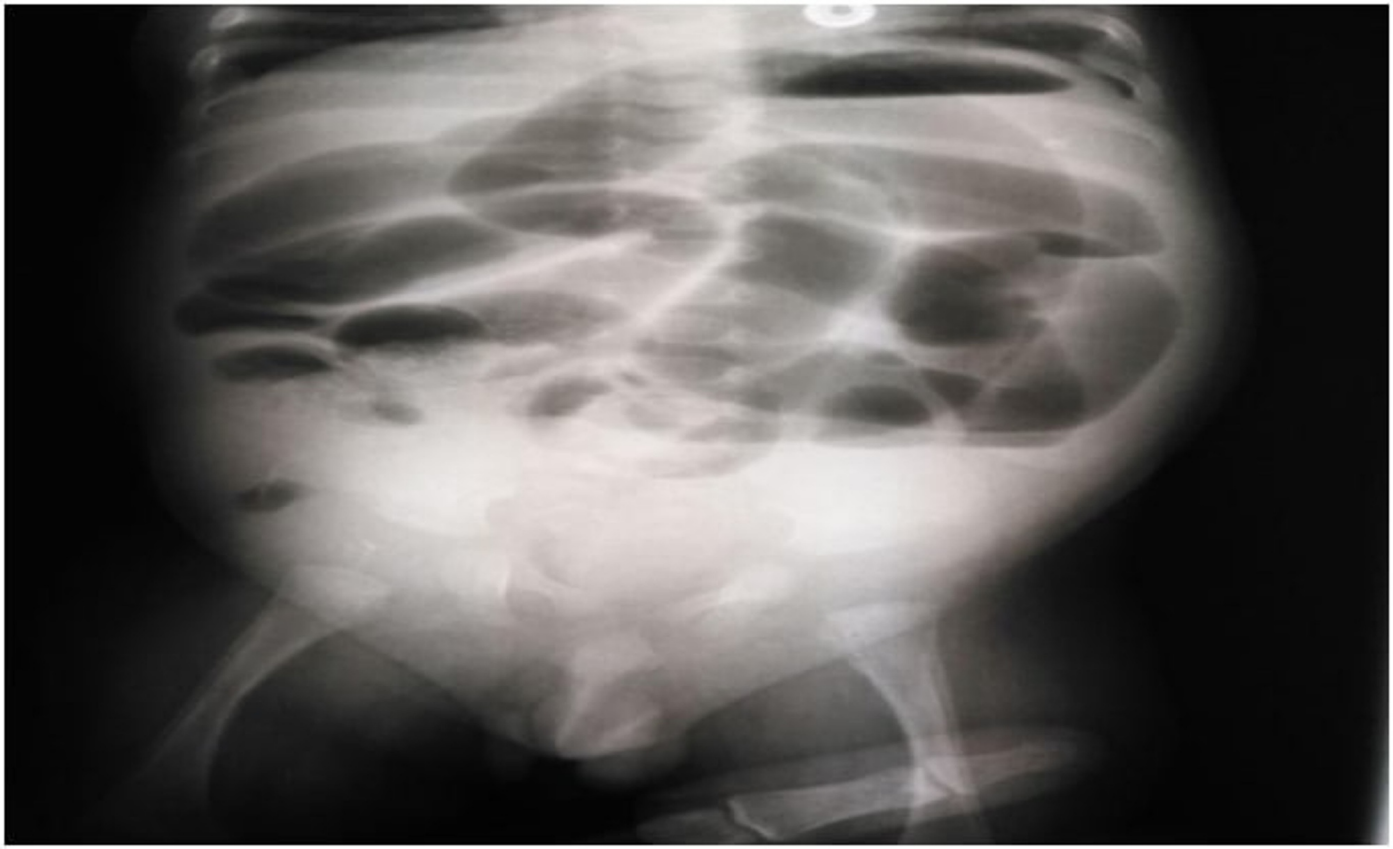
AP supine abdominal radiograph.
